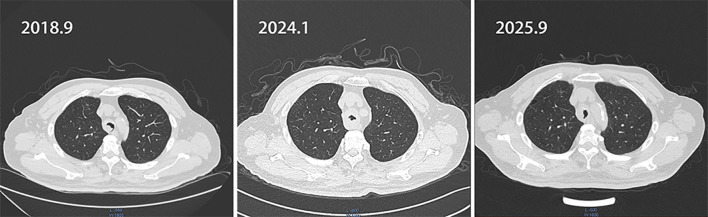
CT indicates recurrent tracheal tumor leading to tracheal stenosis.
